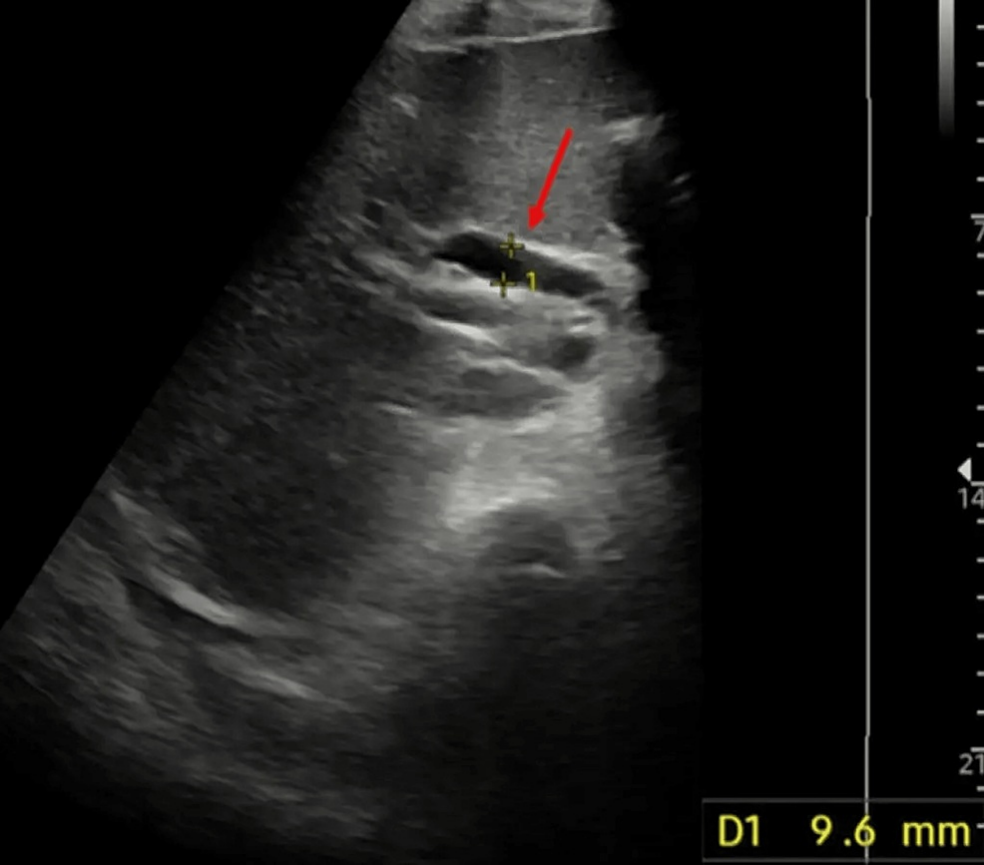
Ultrasound scan of the abdomen with axial grey-scale image showing slightly dilated common bile duct, 9.6 mm (red arrow)
